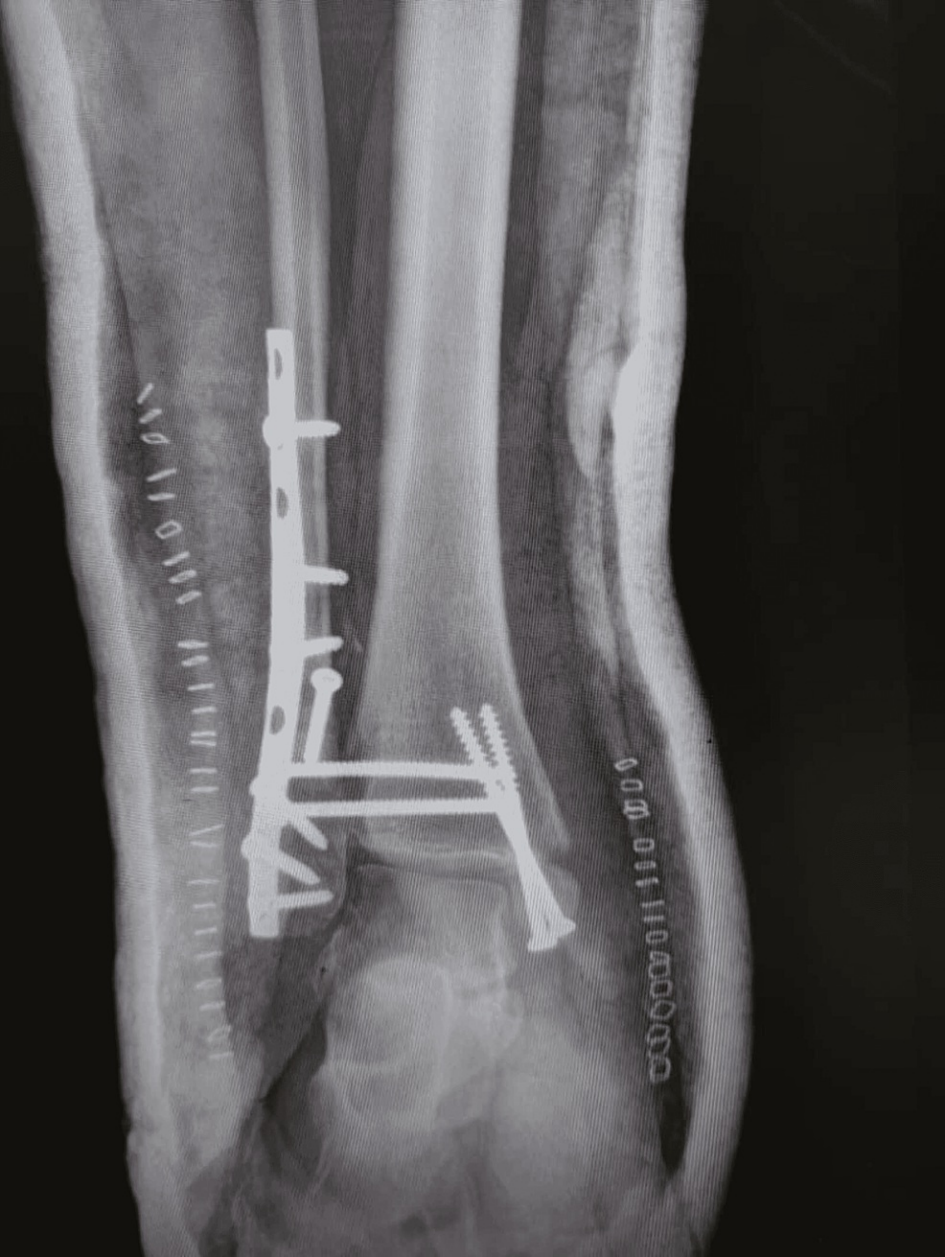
Postoperative radiograph anterior-posterior (AP) view
